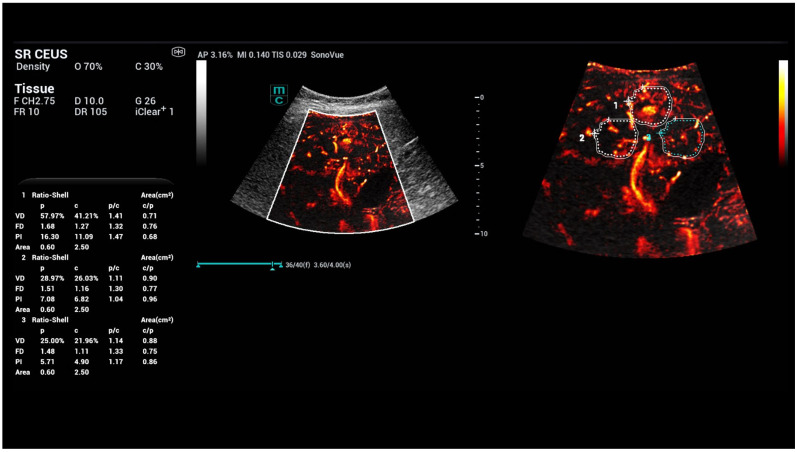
SRCEUS perfusion evaluation after injection of 1.4 mL ultrasound contrast agent with irregular hyperenhancement of the liver and early nodular enhancement of high-flow haemangiomas and micro-shunts. In the early arterial phase, there is strong contrast enhancement in the areas of micro-shunts with capillary changes in the microvascular density and ratio shell (area 1).
